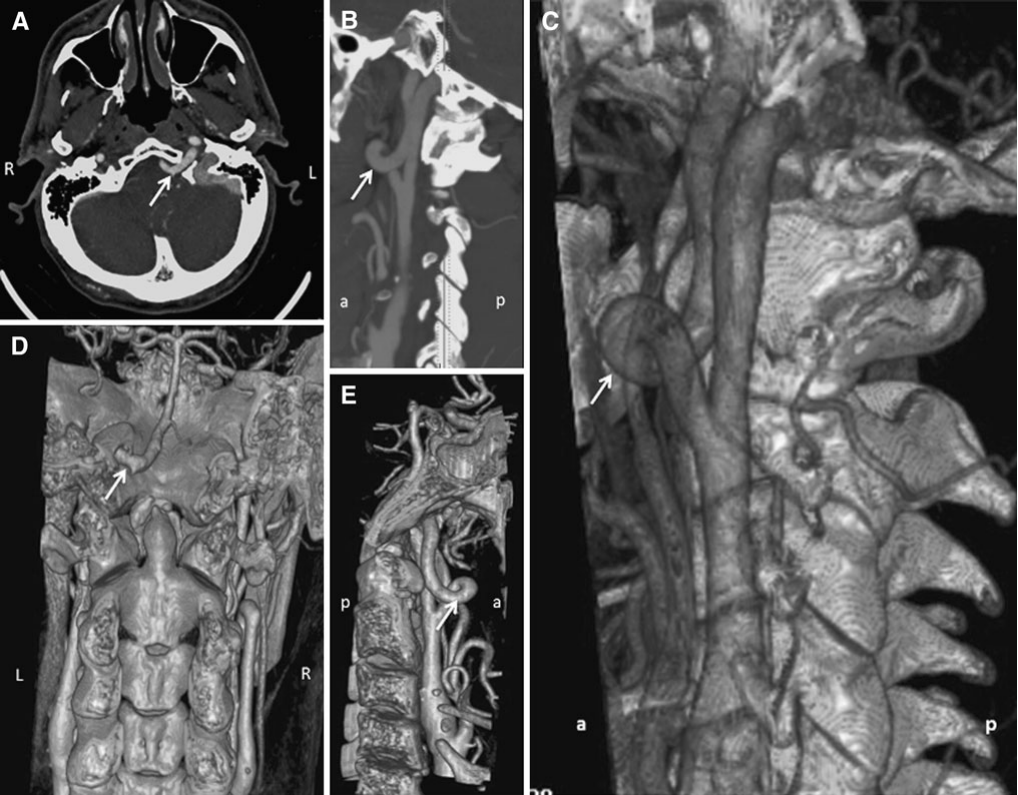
a Axial brain CT-angiography, b, c MIP and 3D-reconstruction CT-angiography showing left PPHA (*arrows*). d Three-dimensional-VR CT-angiography showing PPHA (*arrow*) entering the posterior cranial fossa through an enlarged hypoglossal canal and thus joining the lower portion of the basilar artery, and in e PPHA (*arrow*) as a large vessel originating from the internal carotid artery at the C2 vertebra level
